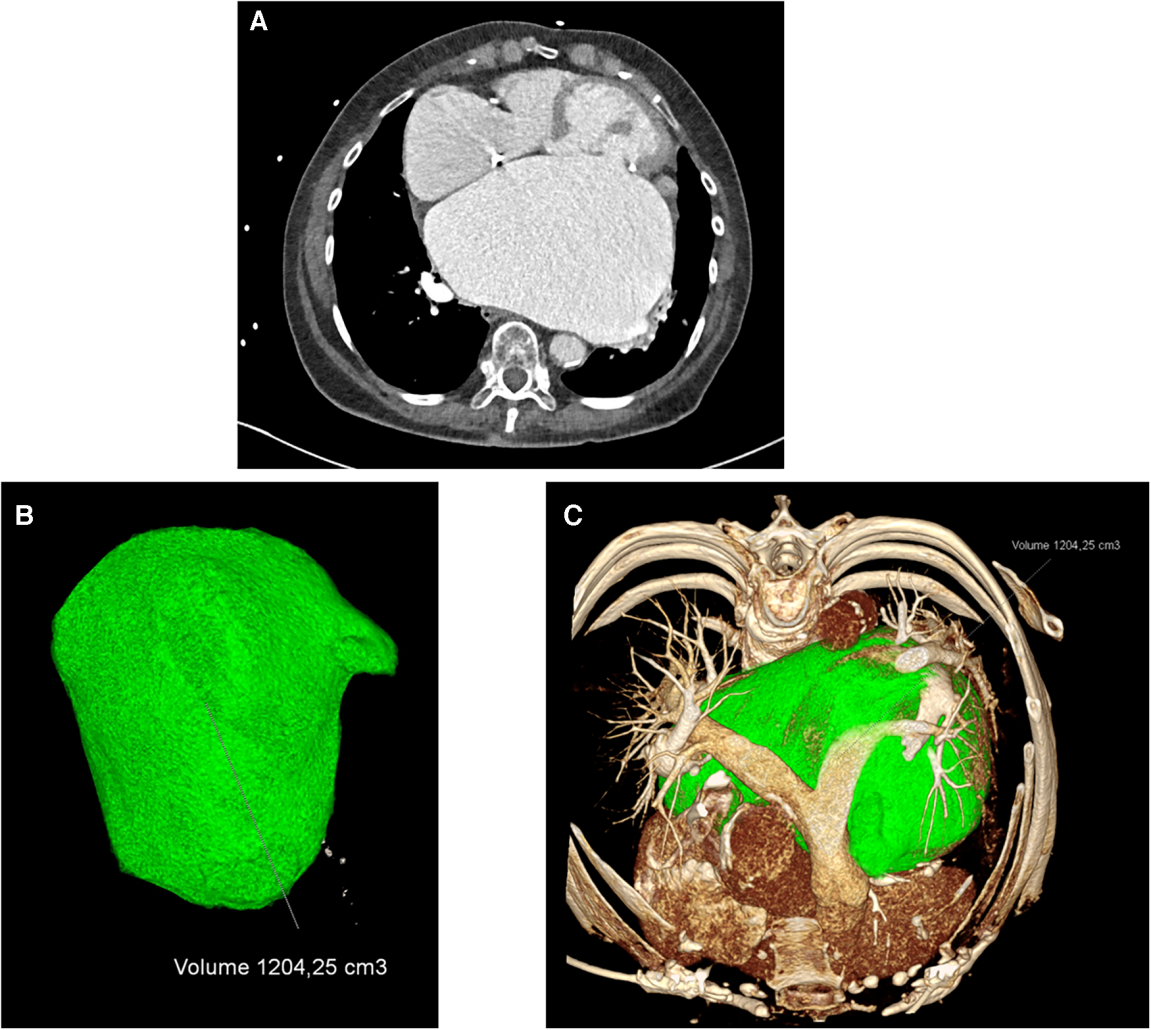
(A) CT scan prior surgery, (B) left atrial 3D volumetric measurements, and (C) left atrial (green) intrathoracic 3D dimensions.
